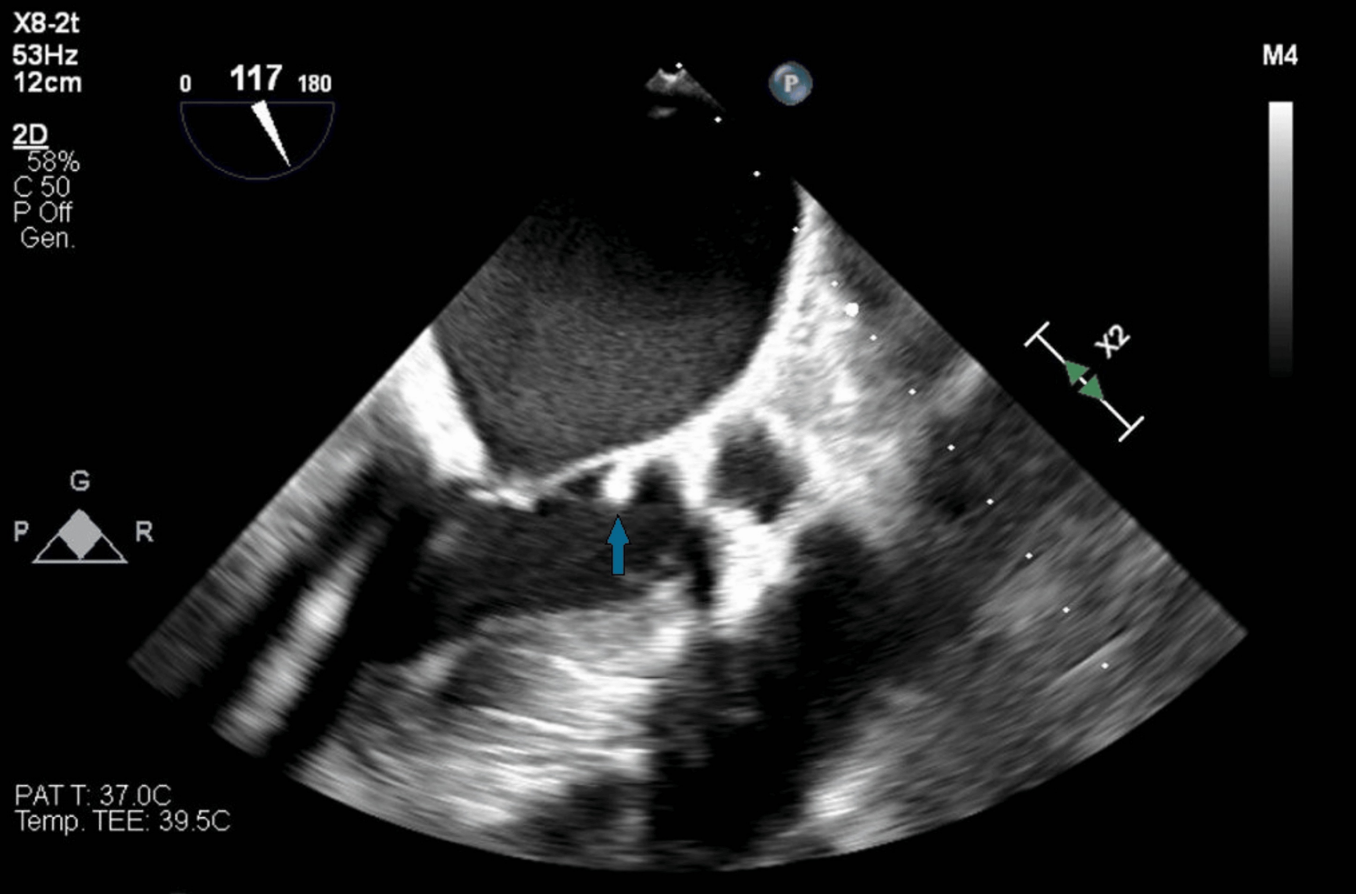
Transesophageal echocardiogram showing vegetation at the mitral-aortic junction.
